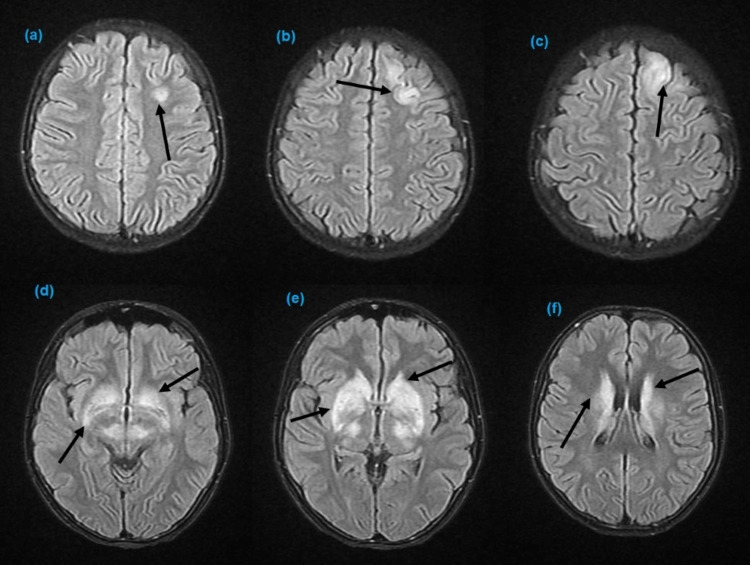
Axial FLAIR T-2 weighted images of the brain. (a–c) hyperintense signals in the left parietal lobe and (d–f) bilateral hyperintense signals in the thalami and corpus striatum. FLIAR: fluid-attenuated invention recovery.
